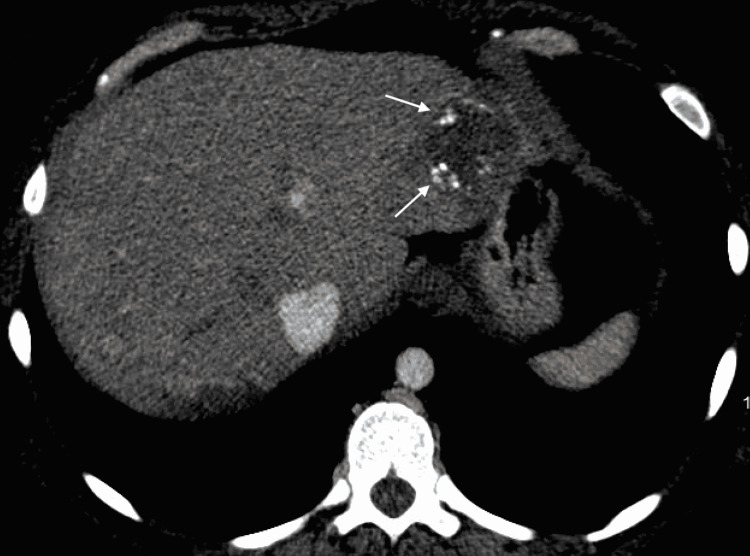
Axial view of a CT abdomen/pelvis showing a hepatic lesion with multiple calcifications present (white arrows) CT: Computed Tomography
